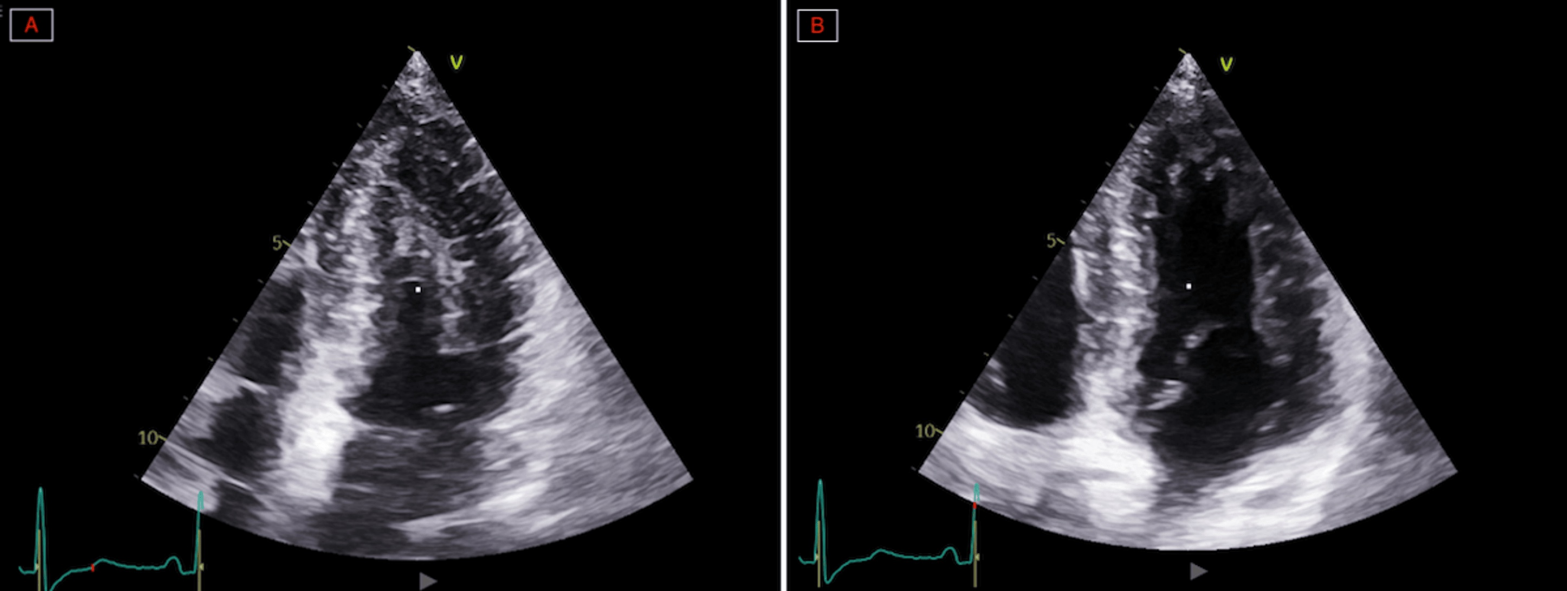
Apical four-chamber transthoracic echocardiographic views focused on the left ventricle on day 3. (A) End-diastolic frame. (B) End-systolic frame. These images demonstrate rapid recovery of left ventricular systolic function, with improvement of left ventricular ejection fraction to 52% and normalization of segmental wall motion.
